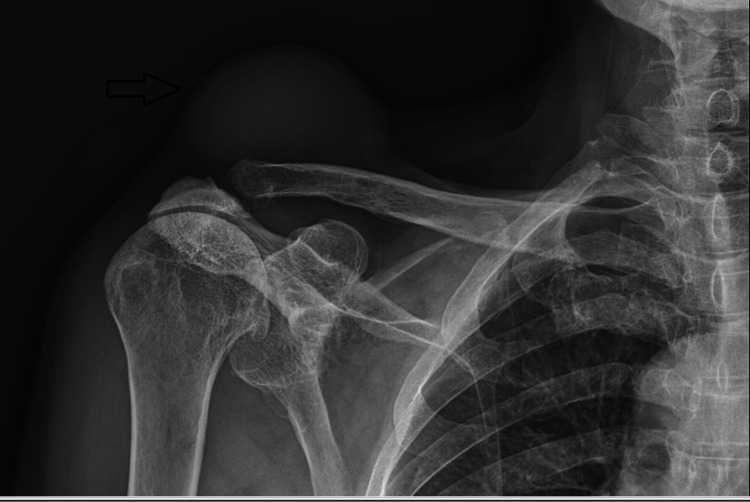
Plain radiograph of the right shoulder showing a soft tissue shadow (black arrow)
